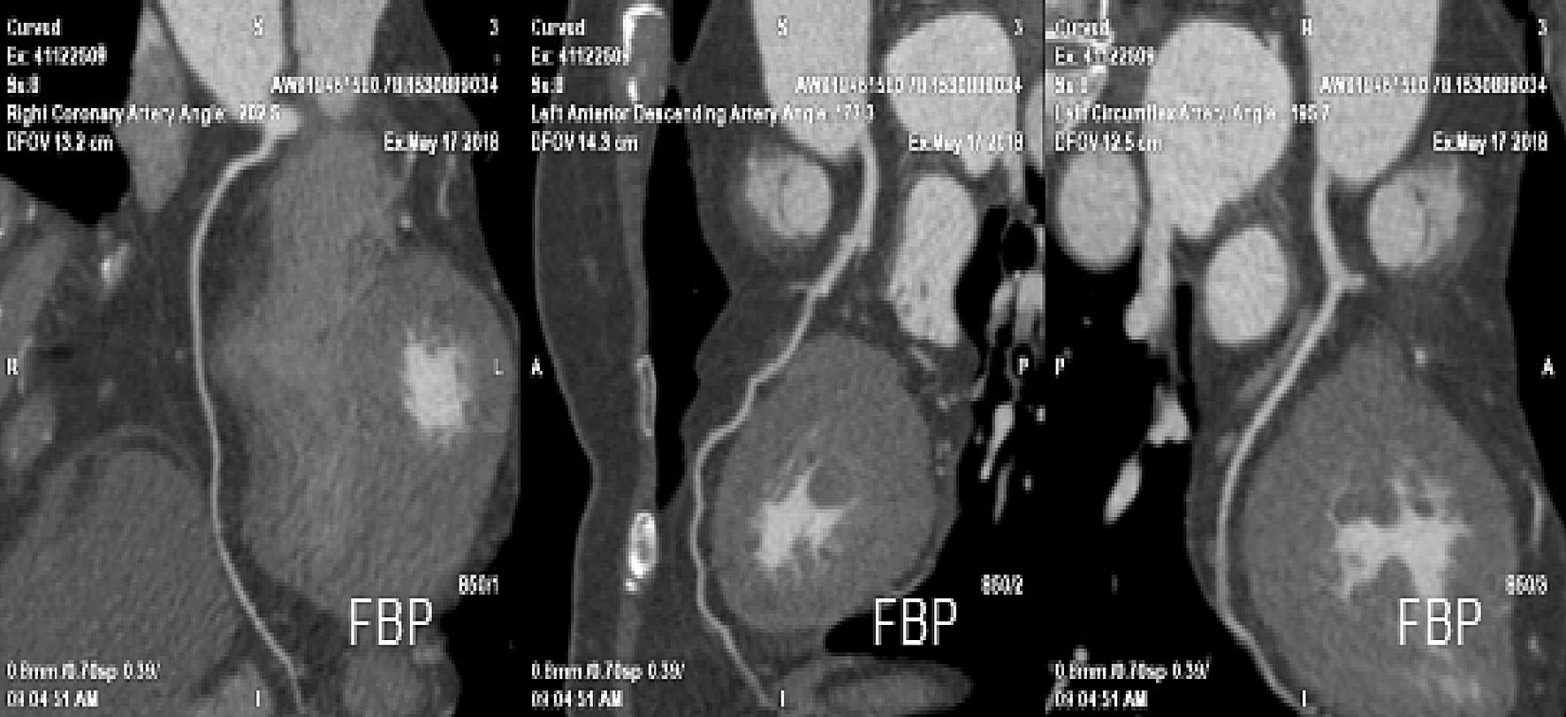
Representative image of a 59-year-old female patient, body surface area (BSA) 1.48 m^2^, body mass index (BMI) 23.8 kg/m^2^, heart rate (HR) 85 bpm, using 40 mL of contrast agent injected at a flow rate of 3.5 mL/s on a Force computed tomography scanner.
